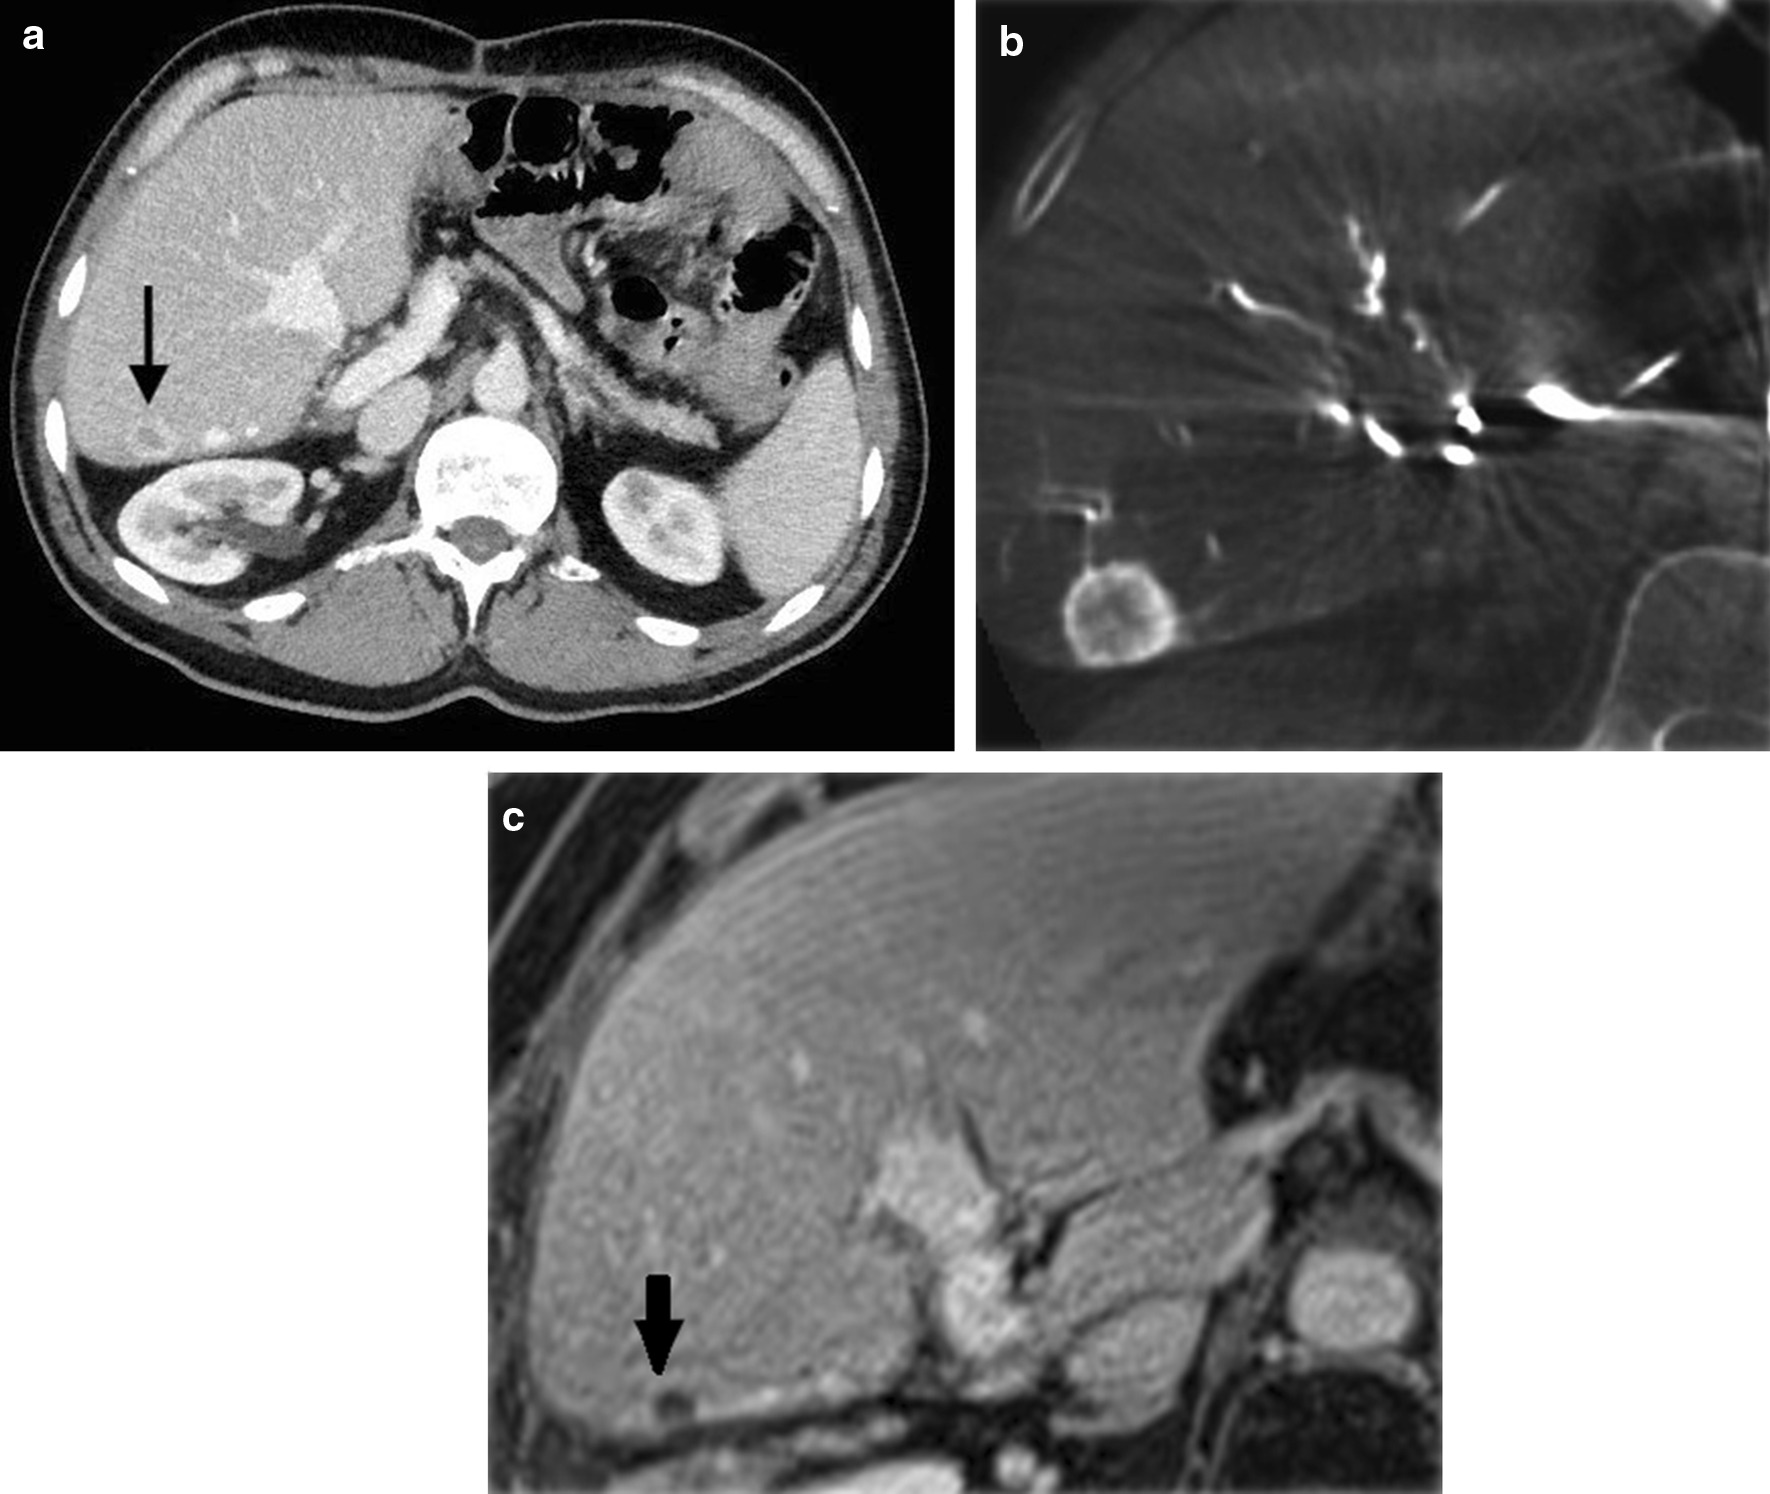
A Axial portal phase contrast-enhanced computed tomography image shows liver metastases in segment VI. B Cone-beam computed tomography prior to superselective Yttrium-90 selective internal radiation therapy confirmed complete uptake of contrast media in the metastases. C Follow-up magnetic resonance image shows complete response according to modified Response Evaluation Criteria in Solid Tumors
